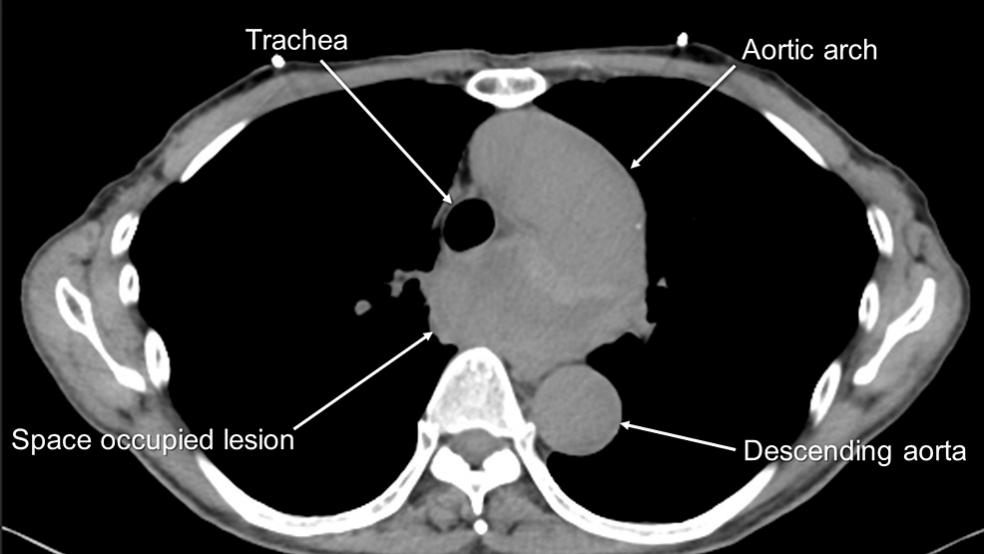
Plain computed tomography (CT) scan demonstrating a space occupying a lesion located posterior to the trachea
